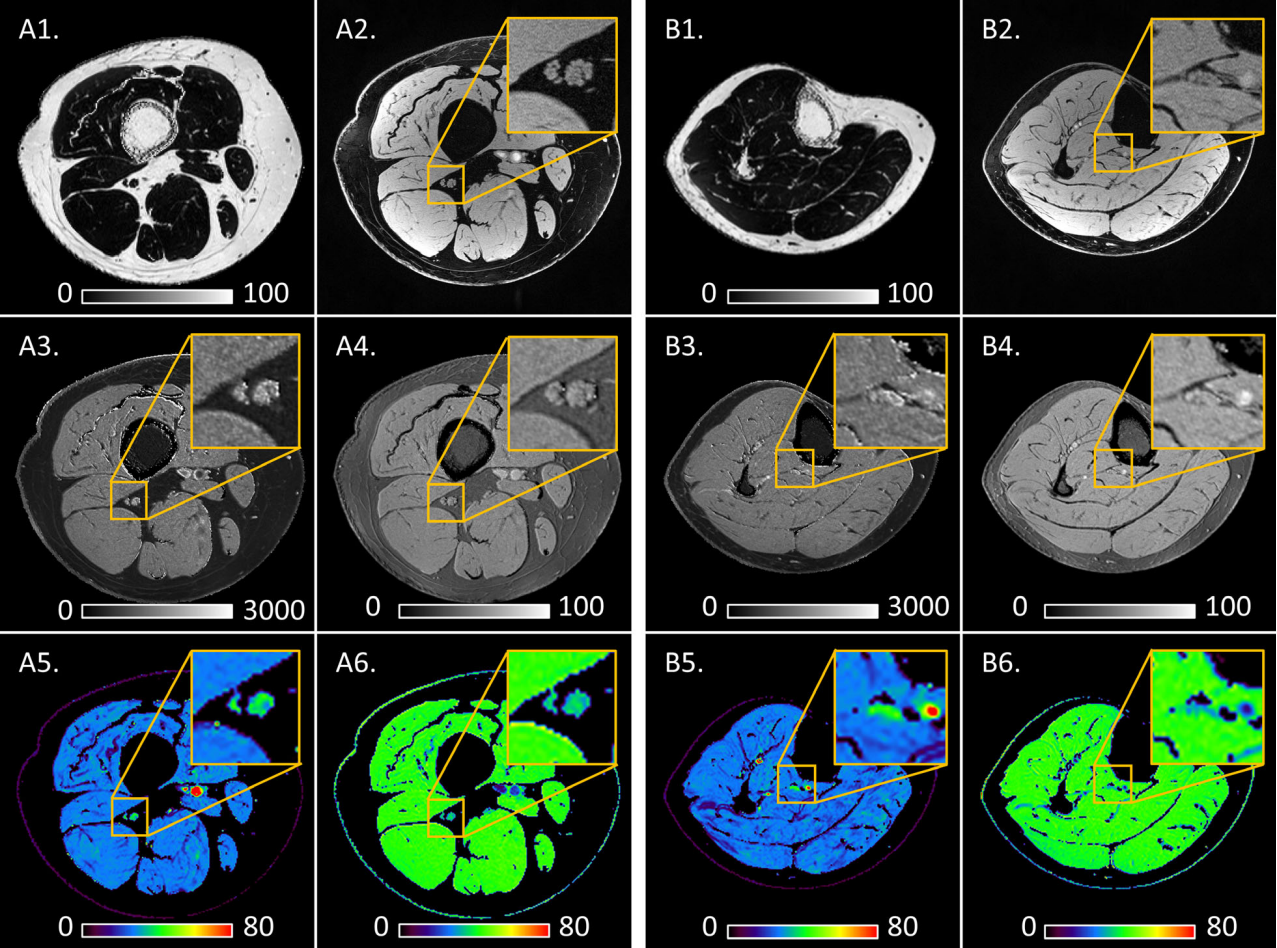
Representative MRI images. These representative data were obtained from a patient with HNPP (52‐year‐old women) at mid‐thigh (panel A) and mid‐calf (panel B) levels. A1 (B1) is thigh (calf) muscle FF image. A2 (B2) is high‐resolution anatomical images for measuring sciatic (tibial) nerve fascicular cross‐sectional area (fCSA). A3 to A6 (B3 to B6) are quantitative images for measuring sciatic (tibial) nerve T1, PD, T2* and MTR, respectively. Sub images on each one were 3x‐zoomed local images on the nerve territories. Images were acquired with 3 mm slice thickness and in‐plane resolution 0.15 x 0.15 mm^2^ for fCSA; 0.3 x 0.3 mm^2^ for T1 and PD; and 0.6 x 0.6 mm^2^ for FF, T2* and MTR. (Units – FF: %; fCSA: mm^2^; T1: ms; PD: a.u.; T2*: ms; MTR: %).
